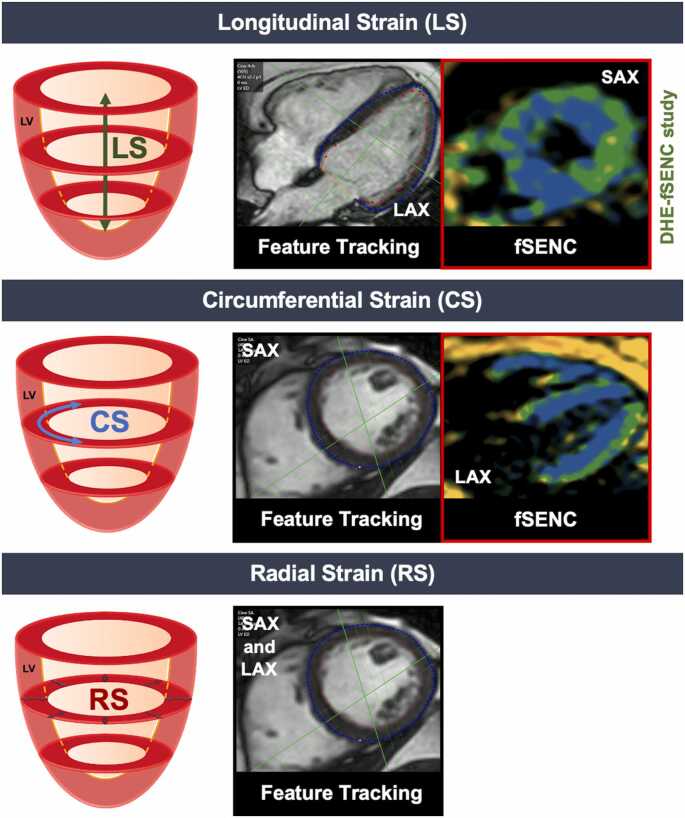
Overview of left ventricular strain directions and their corresponding CMR planes. In contrast to commonly used CMR post-processing tools like feature tracking or speckle-tracking echocardiography, short-axis fSENC slices are required for the assessment of LS, long-axis fSENC slices are required for CS. RS cannot be assessed using fSENC. In this DHE-fSENC study, only LS was assessed. *LS* longitudinal strain, *CS* circumferential strain, *RS* radial strain, *LAX* long axis, *SAX* short axis, *fSENC* fast Strain-ENCoded imaging, *DHE* dynamic handgrip exercise, *CMR* cardiovascular magnetic resonance
